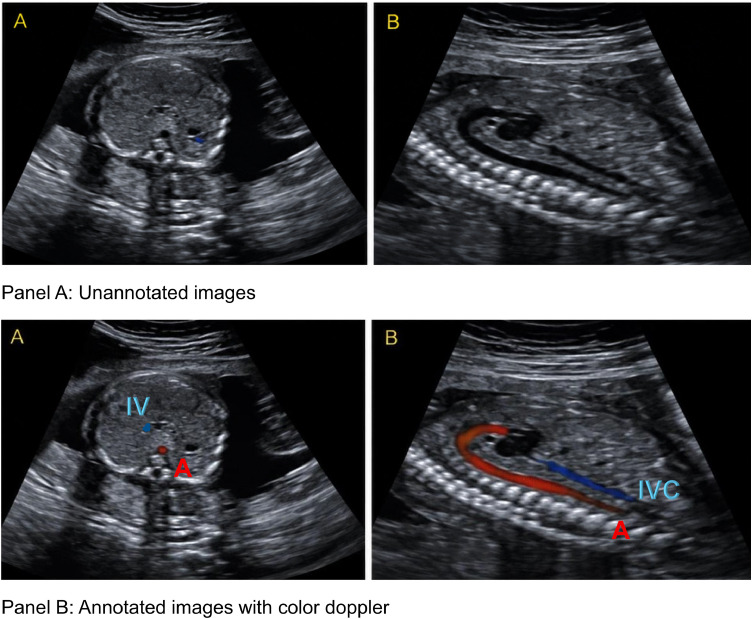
Normal fetal systemic venous anatomy demonstrating the IVC takeoff appearance. Panel A (Unannotated images): axial (A) and sagittal (B) views without annotations. Panel B (annotated images with color Doppler): axial (A) and sagittal (B) views with color Doppler showing the anterior deviation (takeoff) of the inferior vena cava (IVC) from the aorta (AO) after the renal vessels. Abbreviations: AO, aorta; IVC, inferior vena cava.
